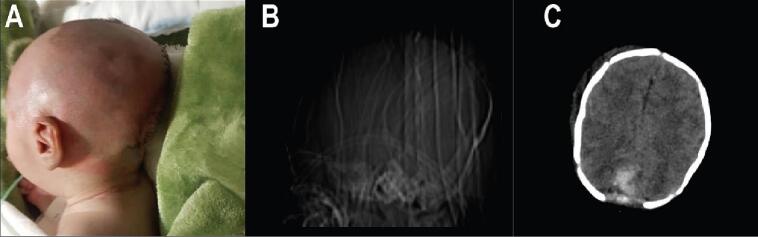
(A) Post-operative photo of the patients. (B) Post-operative plain radiograph showing removal of the lesion. (C) Axial section of the post-operative non-enhanced brain computed tomography scan. There is evidence of post-op change, including occipital bone defect, and also there is a hyperdense area at the site of surgery suggestive of hemorrhage.
